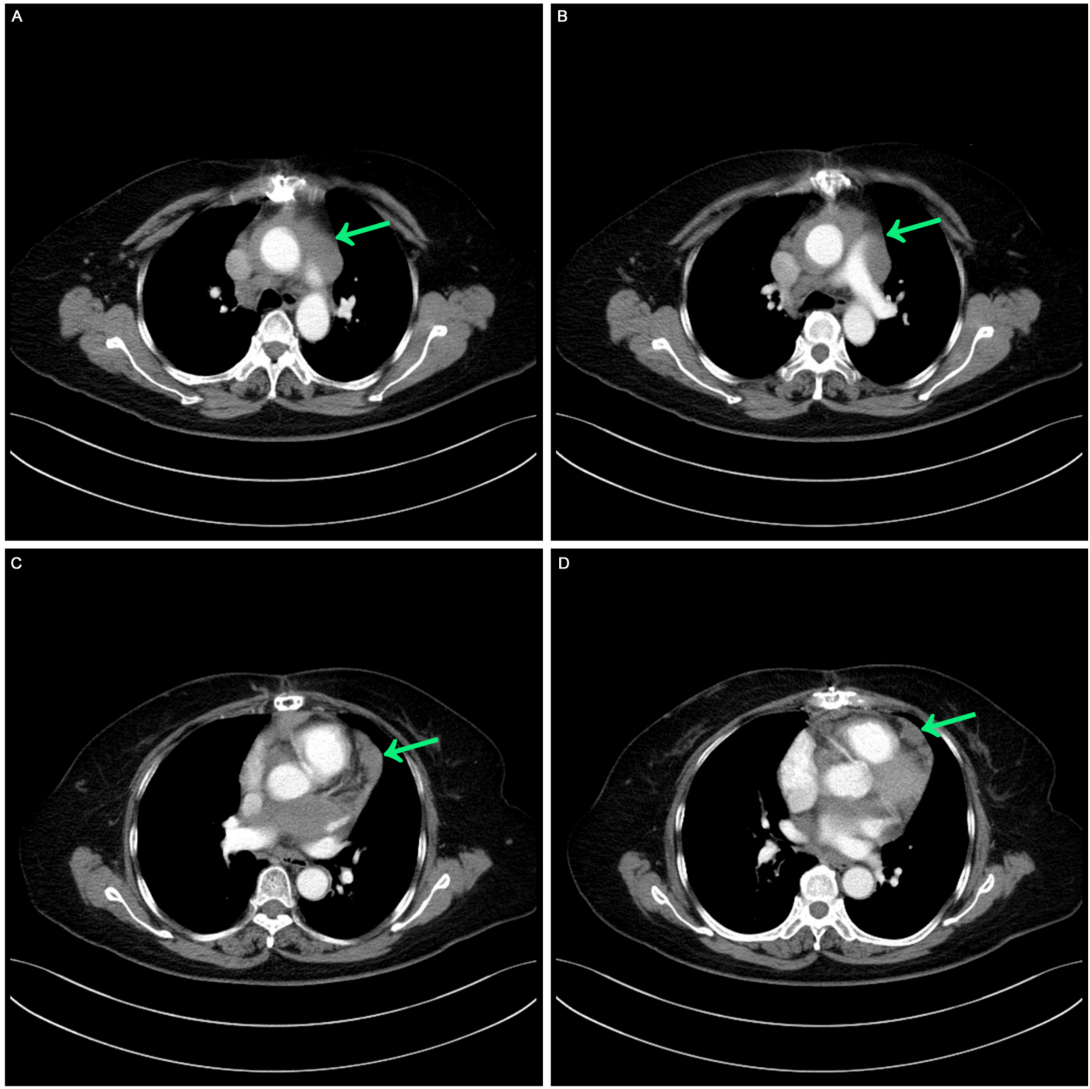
Postoperative contrast-enhanced CT: mild thickening of the pericardium before the third cycle of chemotherapy. (A,B) Computed tomography image showed masses around the aorta and pulmonary arteries. (C,D) Computed tomography image shows mild thickening of the pericardium.
